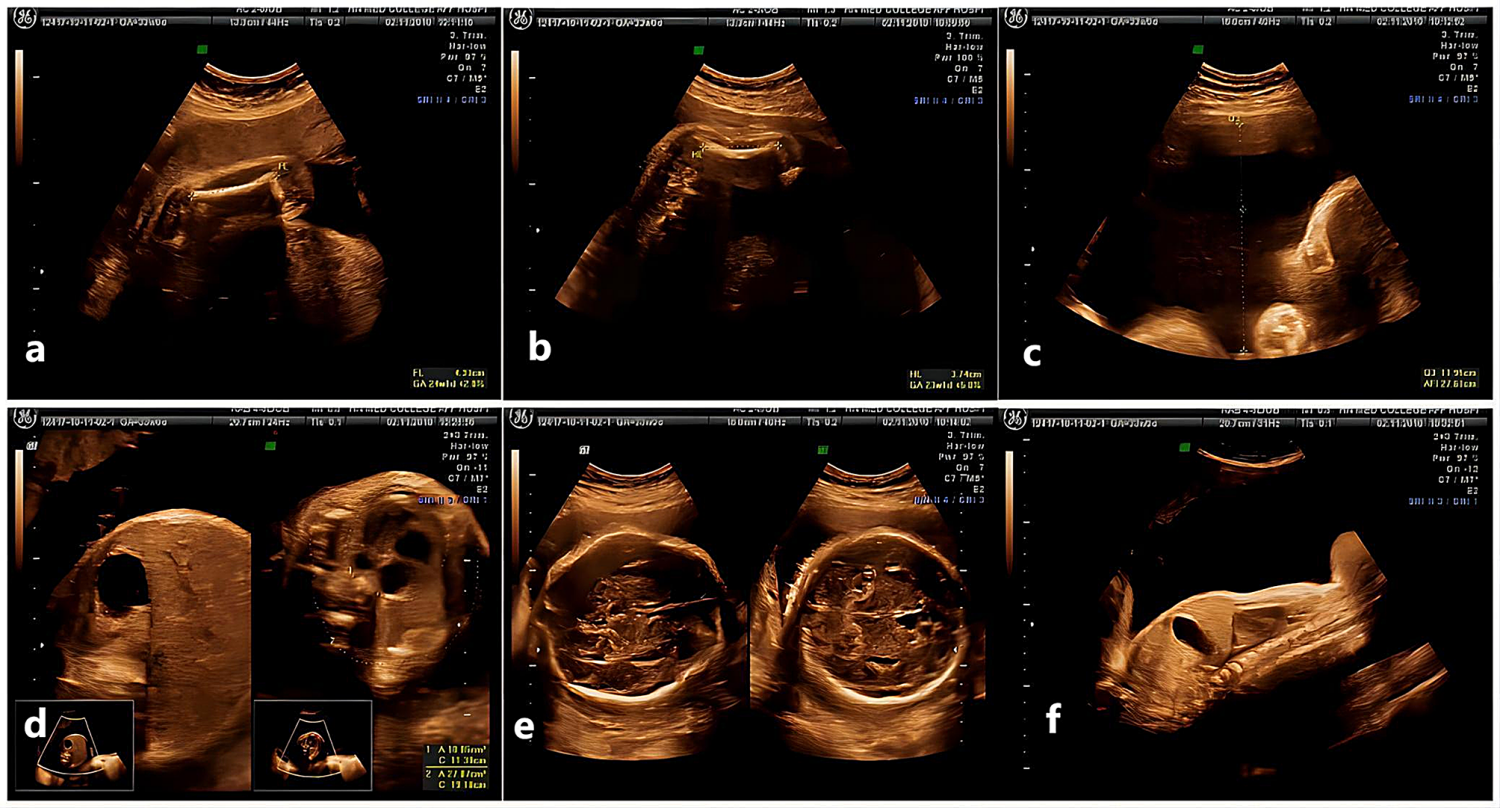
Sonographic features of the second fetus. (a) The FL was equal to 24 weeks of gestation (43 mm). (b) The HL was equal to 23 weeks of gestation (37 mm). (c) Polyhydramnios (depth 12.0 cm and amniotic fluid index (AFI) 44.4 cm). (d) The AC was equal to 29 weeks of gestation (25 cm). (e) The HC was equal to 33 weeks (29.7 cm). (f) Thoracic stenosis (22 cm)
